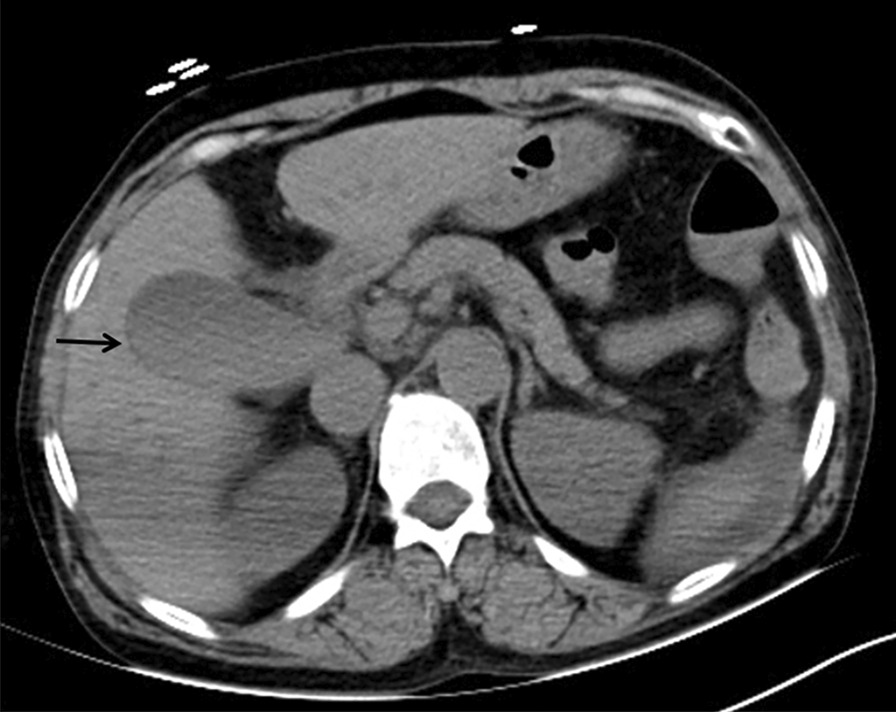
A 54-year-old female with stratification of bile in the lumen. A nonenhanced CT showed stratification of bile (arrow) in the gallbladder. The patient underwent PC the next day, and the bile was black and viscous
